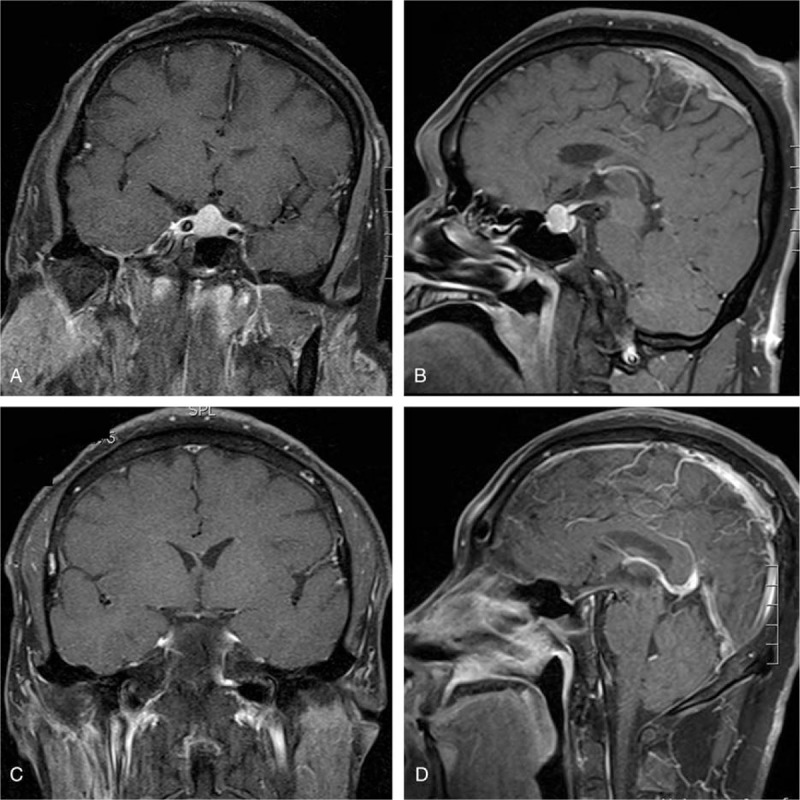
Radiological profiles of Case 2. Intracranial MRI of the patient before treatment (A) coronal and (B) sagittal showing a pituitary mass obviously and uniformly enhanced by Gd-EDTA injection. The upper edge of the mass is round, and optic nerve or optic chiasm were pressed by the mass. (C and D) MRI coronal and sagittal scan showing pituitary return to normal size after taking surgery. MRI = magnetic resonance imaging.
